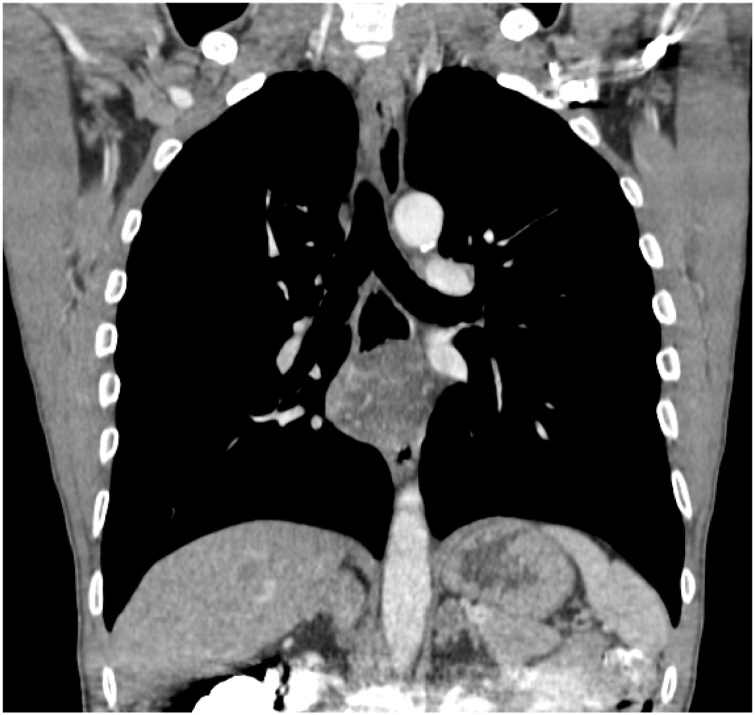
Frontal view of computed tomography of the chest with contrast showing a low oesophagus well-circumscribed mass.
